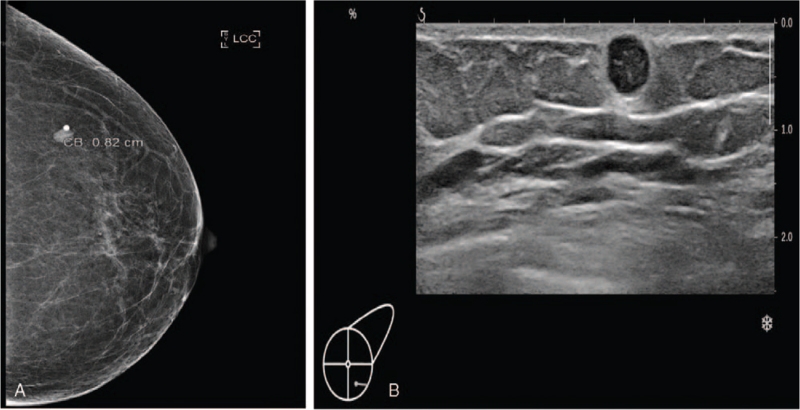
(A) Mammography shows a 0.8 cm circumscribed oval hyper-dense nodule at left breast. (B) Sonography shows a 0.6 cm round hypo-echoic nodule with posterior acoustic shadow at left breast.
